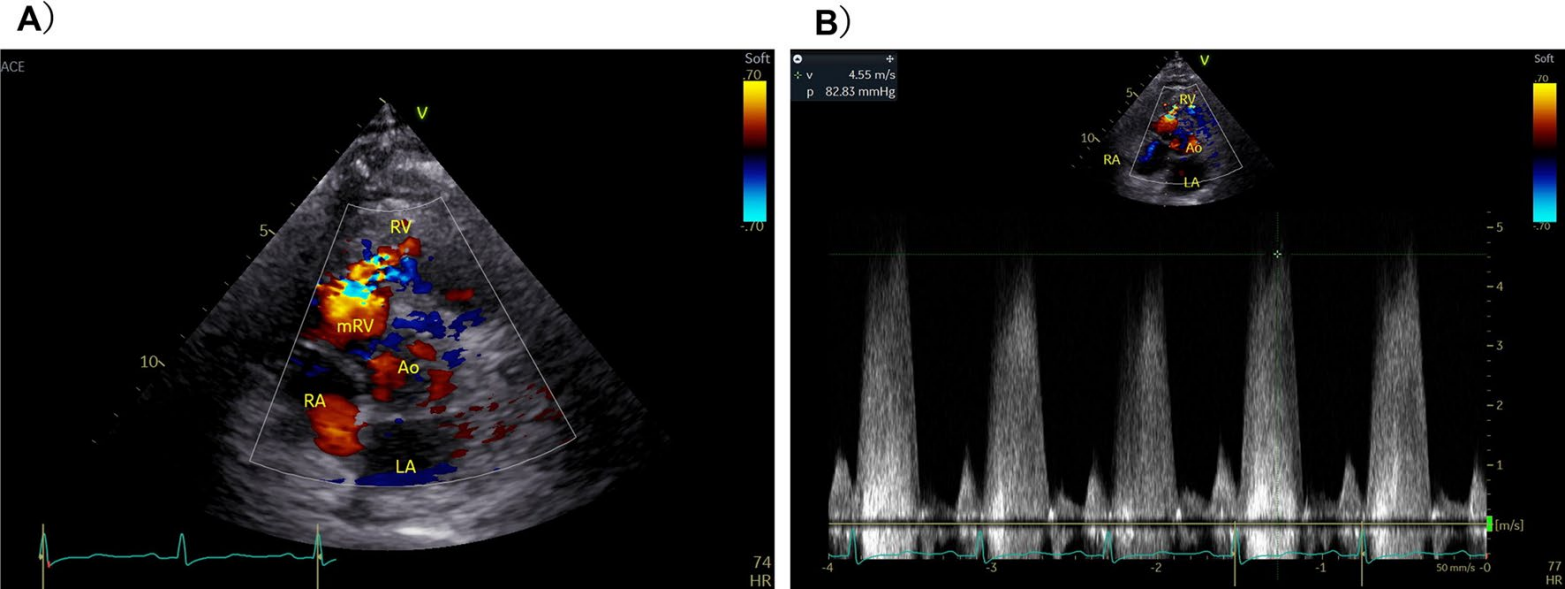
Transthoracic echocardiography revealing a ventricular septum defect in the perimembranous region and right ventricular outflow tract stenosis. A Color Doppler echo showing the short axis view. B Peak pressure gradient was 82 mmHg
